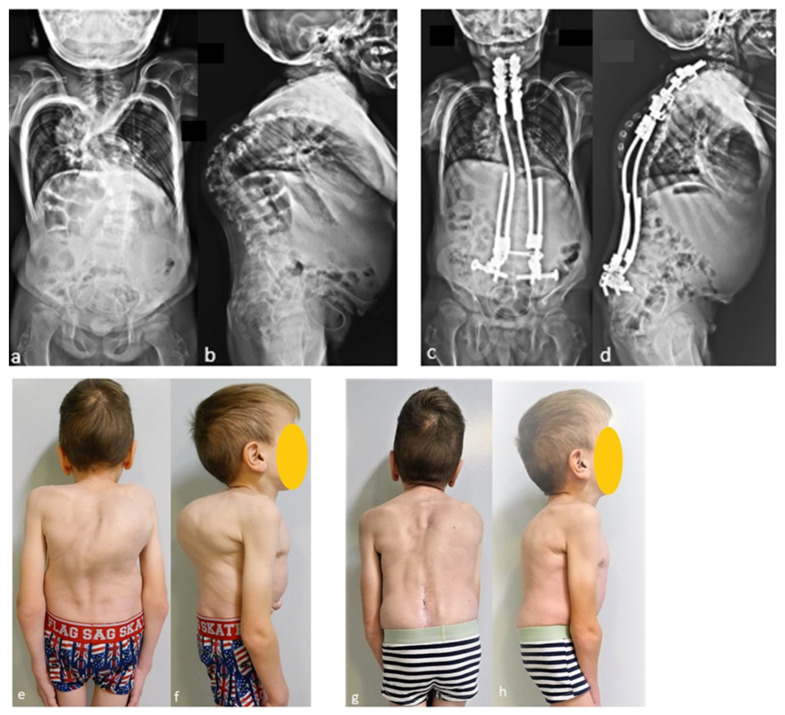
(a–d) Preoperative radiographs and pictures of a 10-year-old boy with kyphosis due to Morquio syndrome; (e–h) 2 year postoperative radiographs and pictures.
